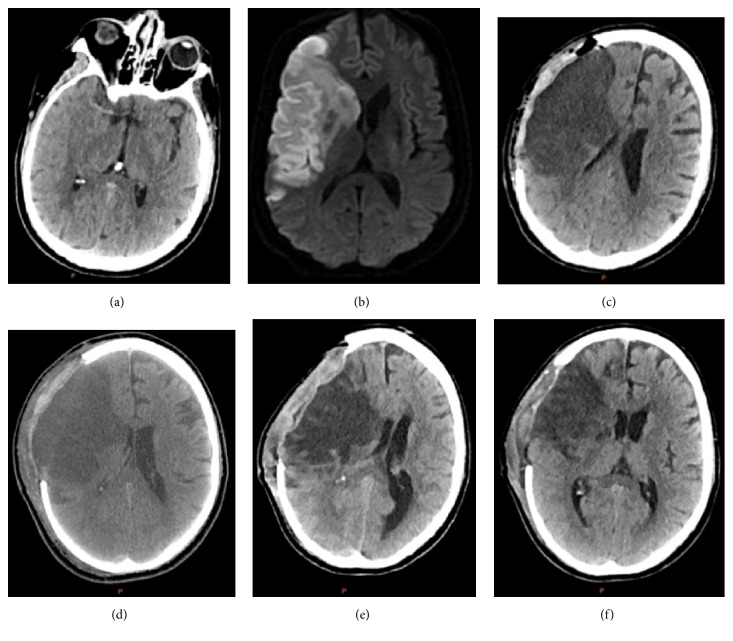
(a) Original CT angiogram revealing a dense right MCA sign. (b) Initial MRI with diffusion weighted sequencing. (c) Postcraniectomy CT. (d) Postop day #4 s/p hemicraniectomy. (e) Sunken flap CT, POD #8. (f) Head CT postop day #12.
